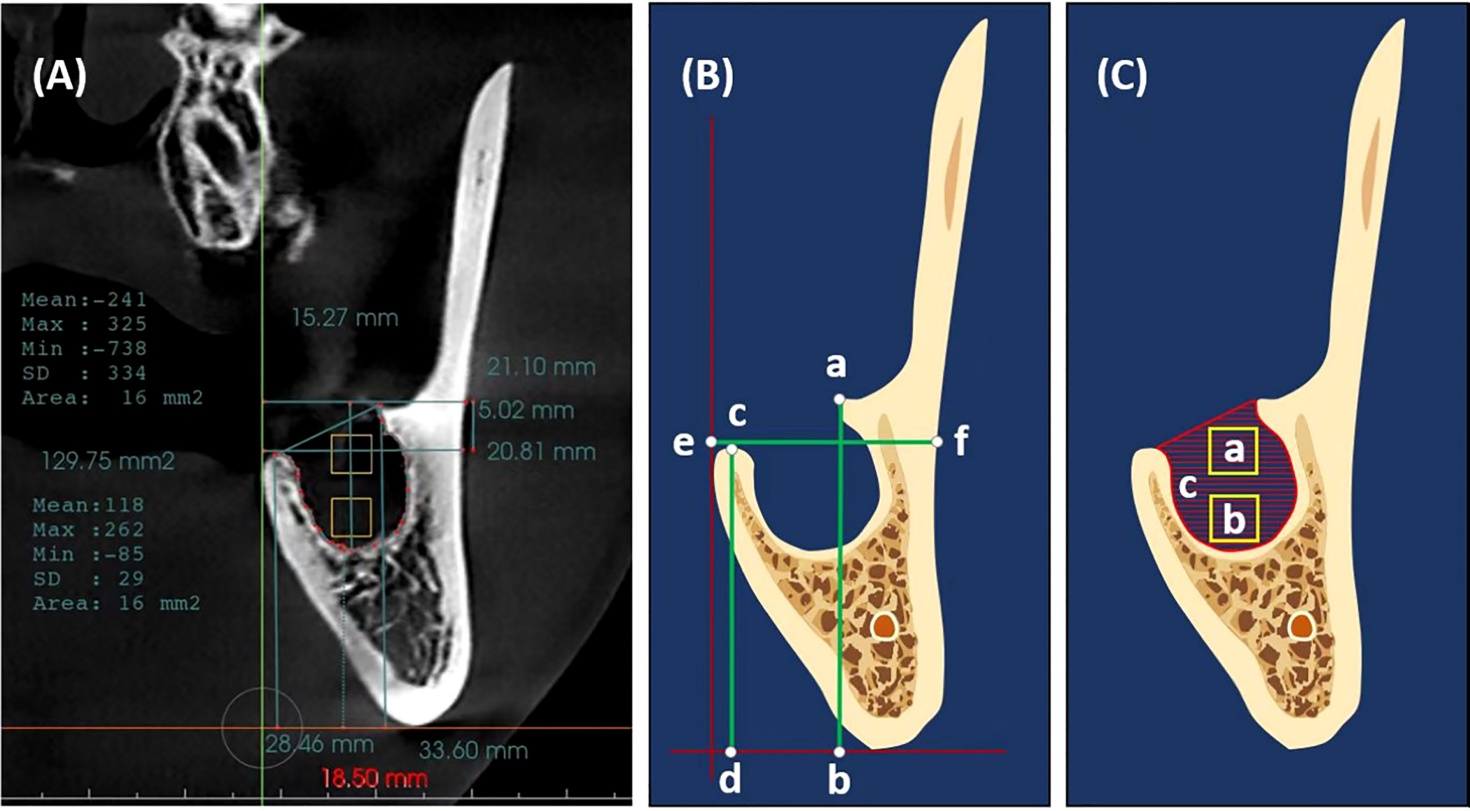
Measurements methodology (A) A cone beam computed tomography (CBCT) scan immediately after extraction with Invivo Dental showing the measurement methodology. (B) Measurements of buccal alveolar bone height (a, b), lingual alveolar bone height (c, d), and alveolar bone width (e, f). (C) Measurements of bone density (a, b squares in the coronal and apical halves of the extracted socket) and socket surface area (c, Hatch area); the outline of the remnant socket.
